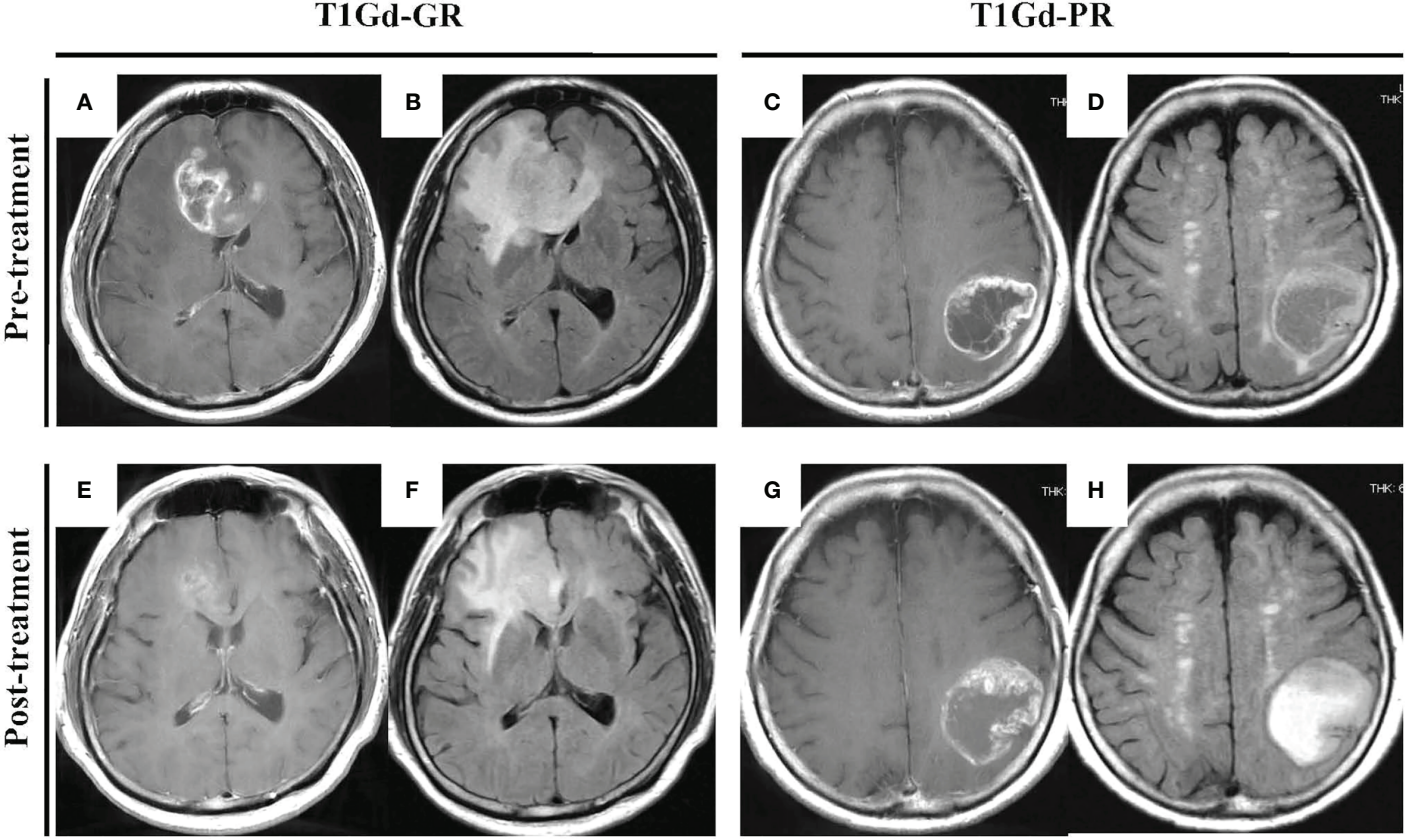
“Typical” GBM demonstrating a huge enhanced tumor with perifocal edema before (A–D) and after (E–H) neoBev. Regression rates of T1Gd-GR (A, E), T1Gd-PR (C, G), FLAIR-GR (B, F), and FLAIR-PR (D, H) after neoBev were −61%, −14%, −71%, and −26%, respectively. FLAIR, fluid-attenuated inversion recovery; GBM, glioblastoma; GR, good rensponder; PR, poor responder; T1Gd, T1-weighted images with gadolinium enhancement.
